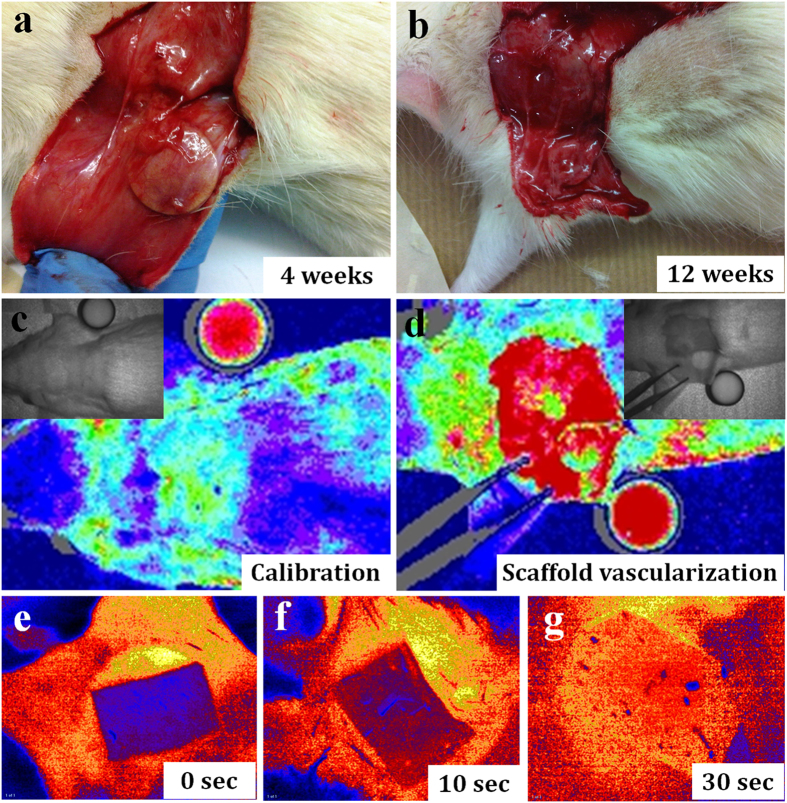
Macroscopic view of subcutaneously implanted scaffolds at (a) 4 weeks and (b) 12 weeks. (c) Laser Doppler calibration using full fat milk and (d) analysis of vascularized scaffolds prior to explantation. (e–g) Monitoring of oxygen transfer from scaffolds through an oxygen sensor film as a measure of scaffold vascularization.
